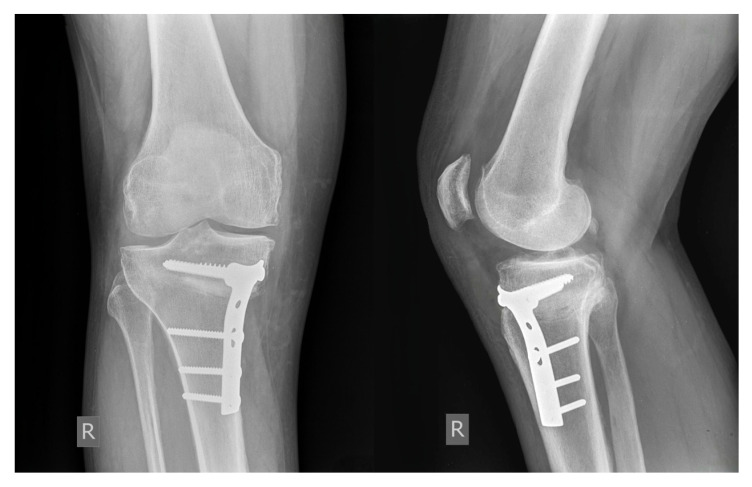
Anteroposterior and lateral radiographs after a MOWHTO using a cancellous bone allograft and fixation with a T-profile AO plate and screws (Shimadzu CH-200, Kyoto, Japan).
